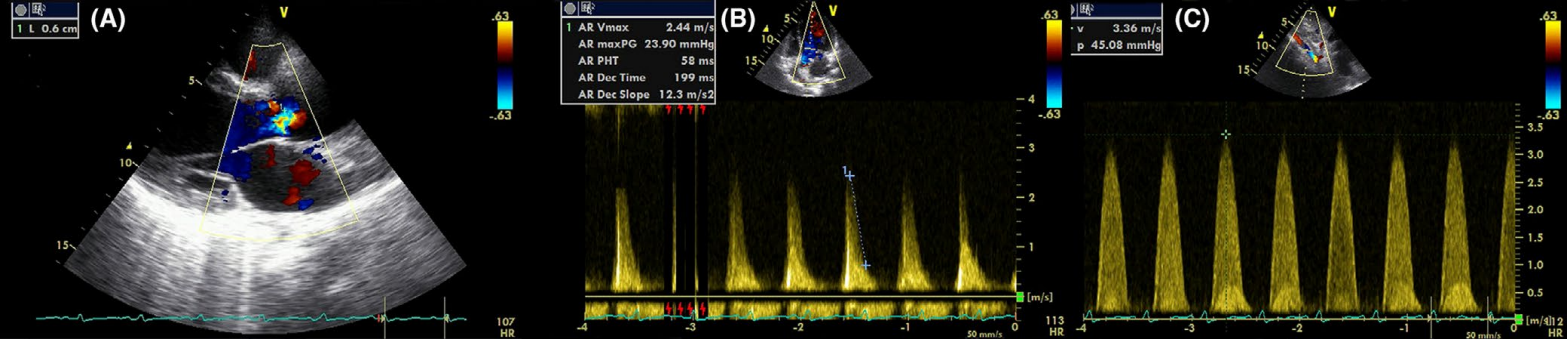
(A) Color Doppler image at parasternal long axis showing vena contracta of 6 mm aortic regurgitation (B) continuous‐wave Doppler of aortic regurgitation showing short pressure half time of 58 ms (C) Continuous‐wave Doppler echo showing increased velocity 3 m/s at the abdominal level
